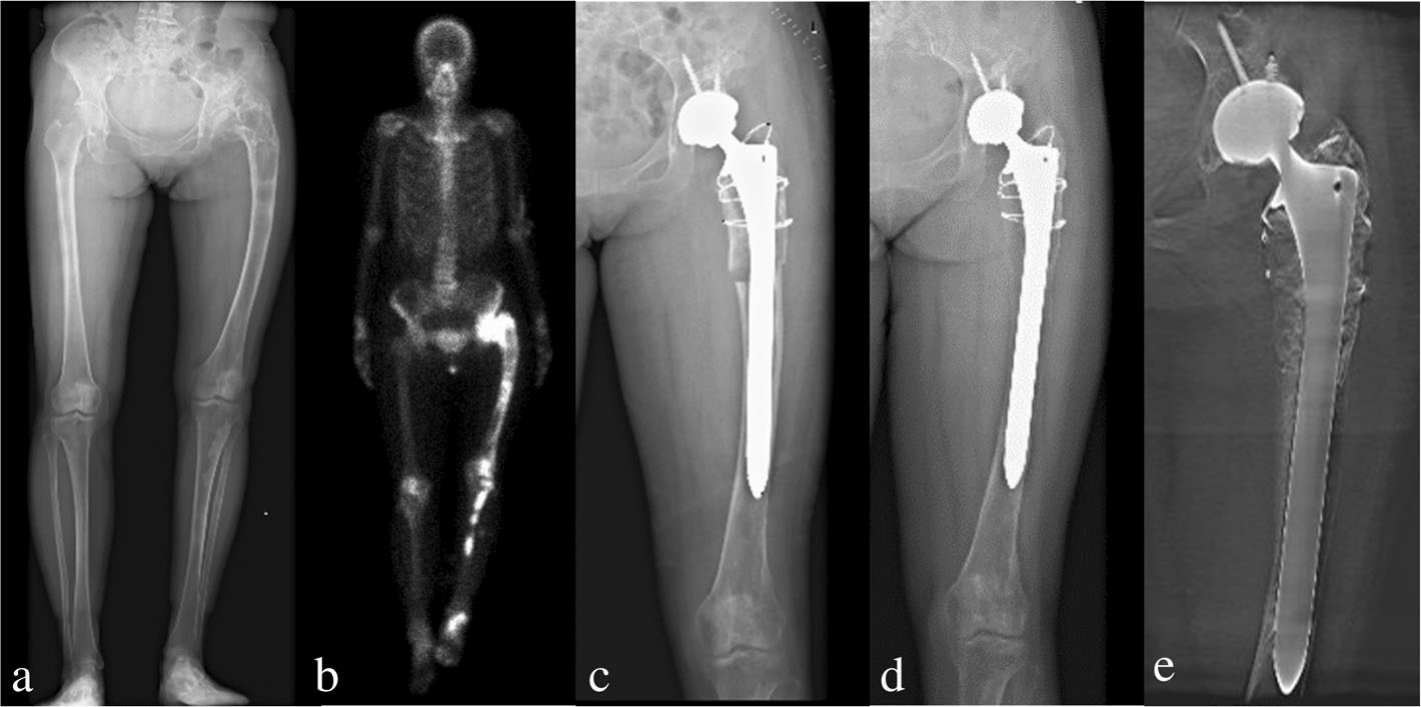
Preoperative radiography (a), bone scintigraphy (b), postoperative radiography (c), and at the 18 months follow-up of radiography (d) and T-SMART image (e) in patient 1
